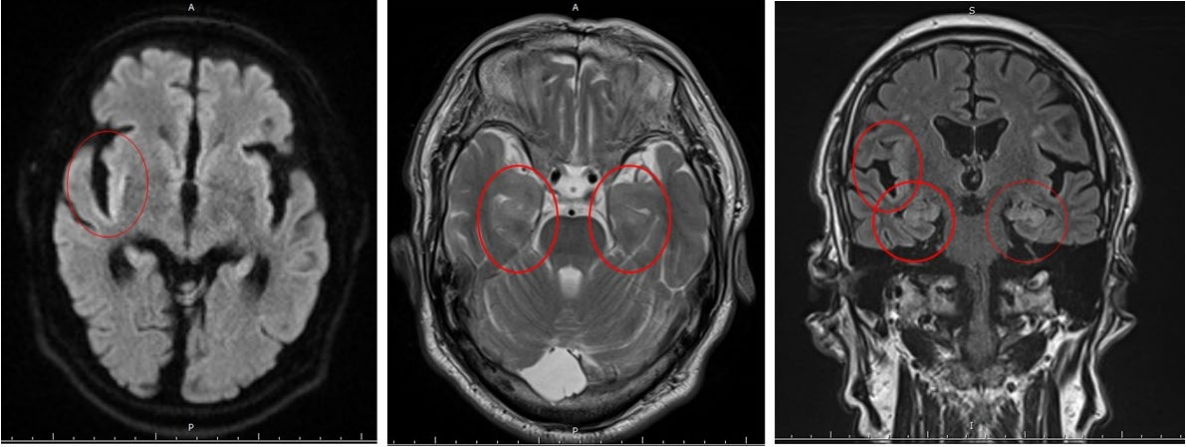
Diffusion‐weighted (left), T2 (middle), and T2‐FLAIR (right) MRI. MRI showed bilateral swelling of the medial temporal lobes and hippocampi and high signal on T2‐weighted imaging; diffusion‐weighted imaging showed high signal predominantly in the right insular cortex; and T2‐FLAIR also showed high signal predominantly in the right insular cortex. In addition to the insular cortex, T2‐FLAIR also showed a high signal in the hippocampus
